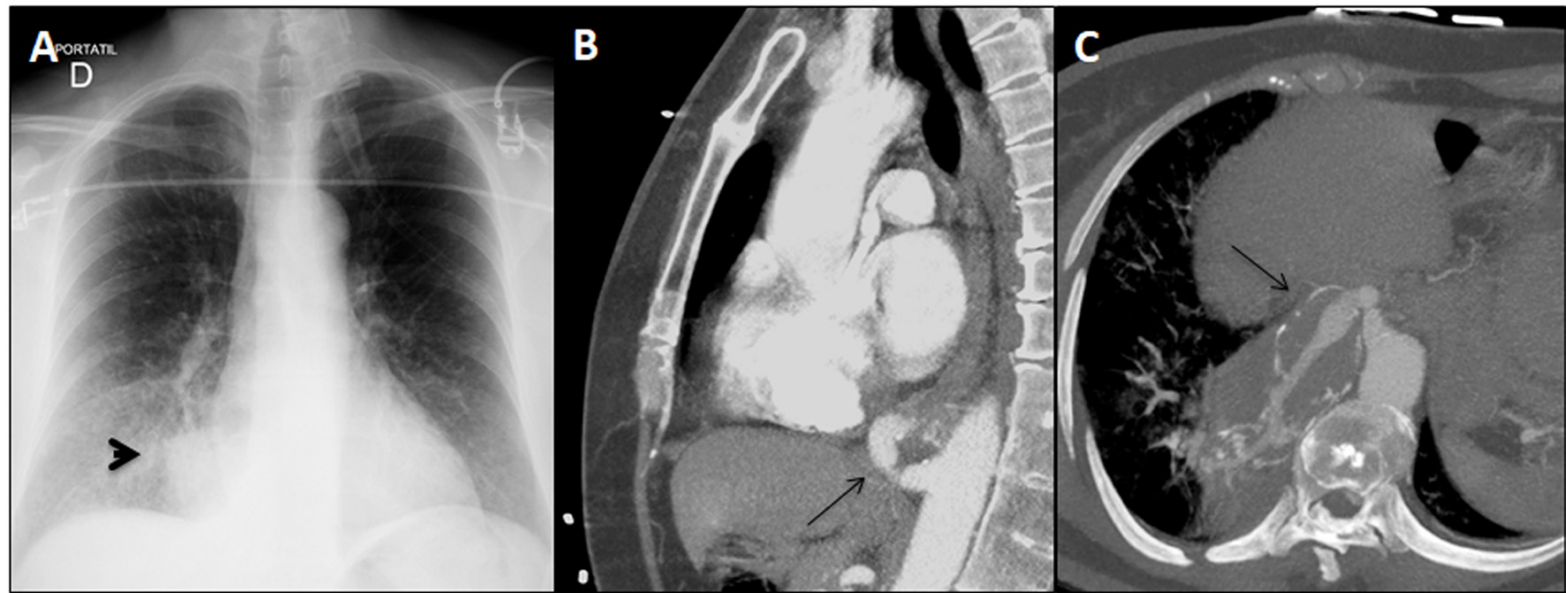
Initial imaging tests. (A) Parenchymal opacity on Chest X-ray (arrowhead); (B) and (C) Origin of aberrant aneurysmal artery with calcified wall and mural thrombus supplying abnormal pulmonary parenchyma on CTA (black arrows).
